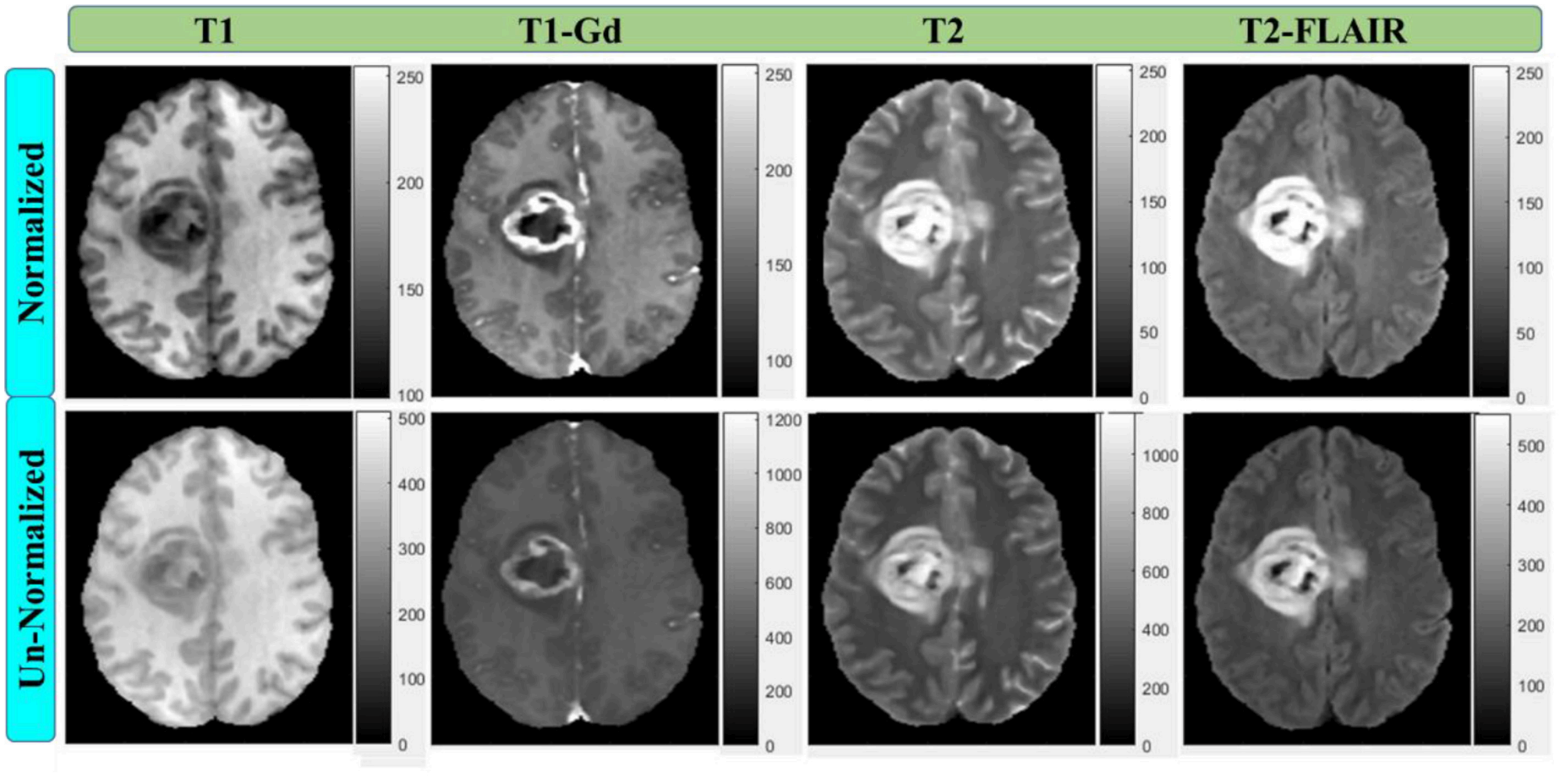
Multi-parametric MRI sequences before and after intensity normalization with 256 scale (8-bit depth). All normalized images have the same scale.
